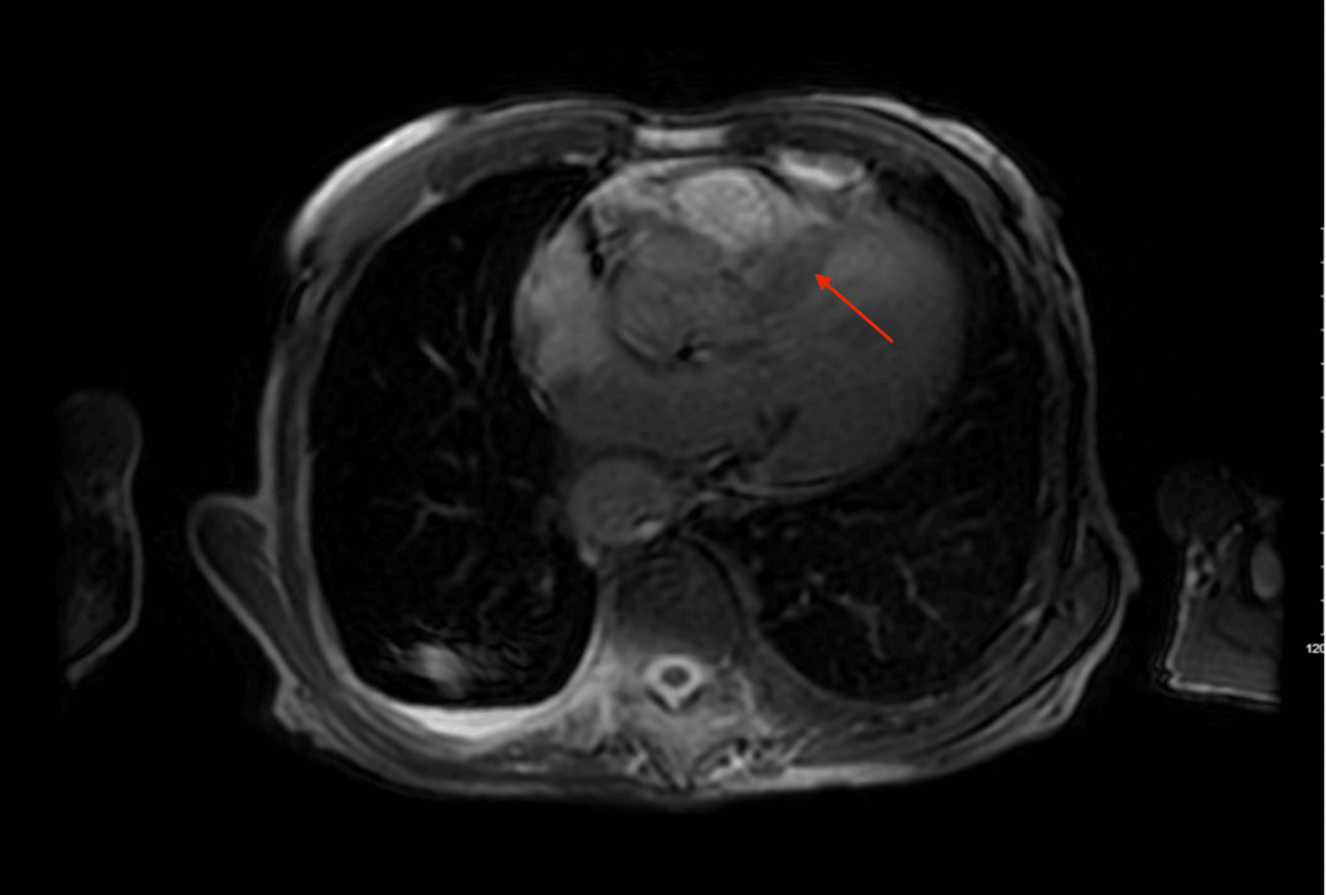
Cardiac MRI (axial view) showing a mass (red arrow) attached to the mitral annulus
